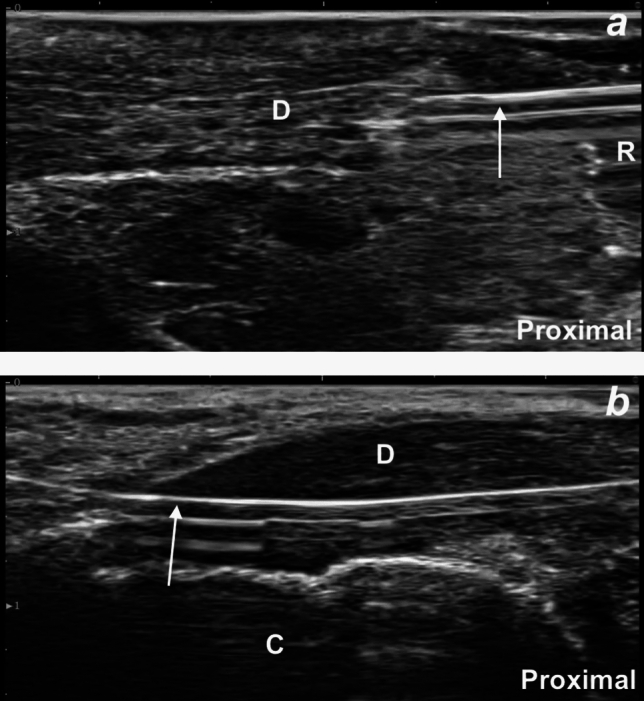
a, b Ultrasound images showing the needle advancing through the distal end of the Achilles tendon (a) and the needle exiting the tendon (b). Arrow: needle, C: calcaneus, D: distal end of the tendon, R: rupture site
